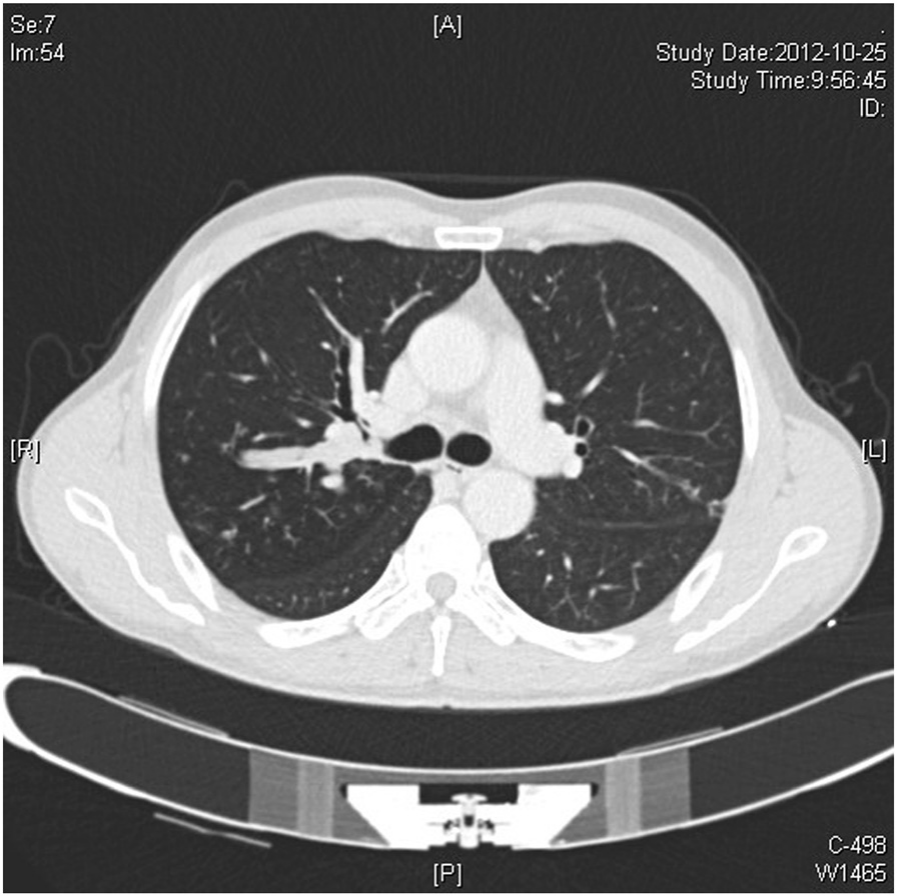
A computed tomographic image had shown the tumor originating from the right upper lobe bronchus (lung windows).
